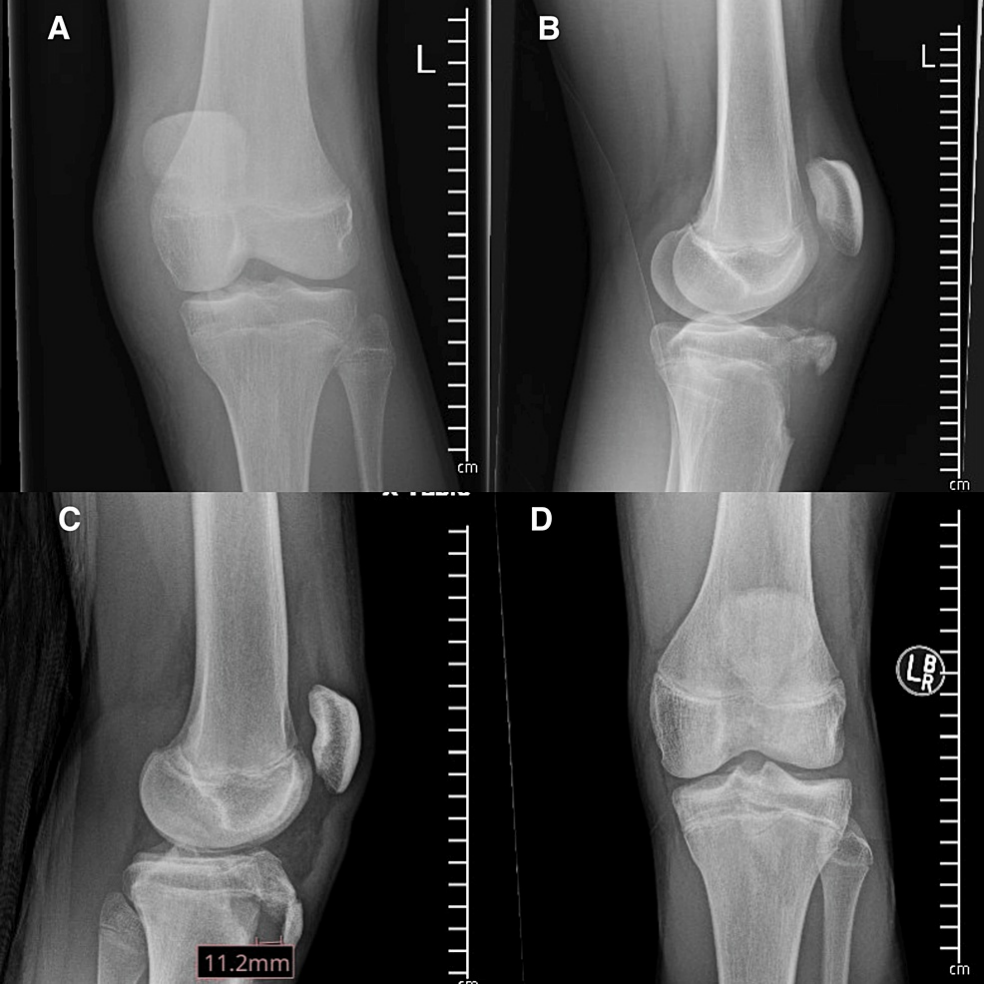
Injury radiographs from case patients: (A) AP radiograph of the knee in patient 1; (B) lateral radiograph of the knee in patient 1; (C) AP radiograph of the knee in patient 2; and (D) lateral radiograph of the knee in patient 2. AP, anteroposterior
